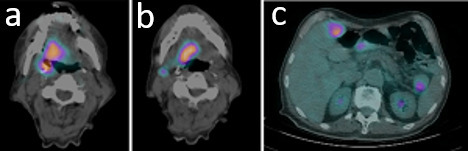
Non-contrast positron emission tomography CT at the site of head and neck cancer and liver metastasis.
